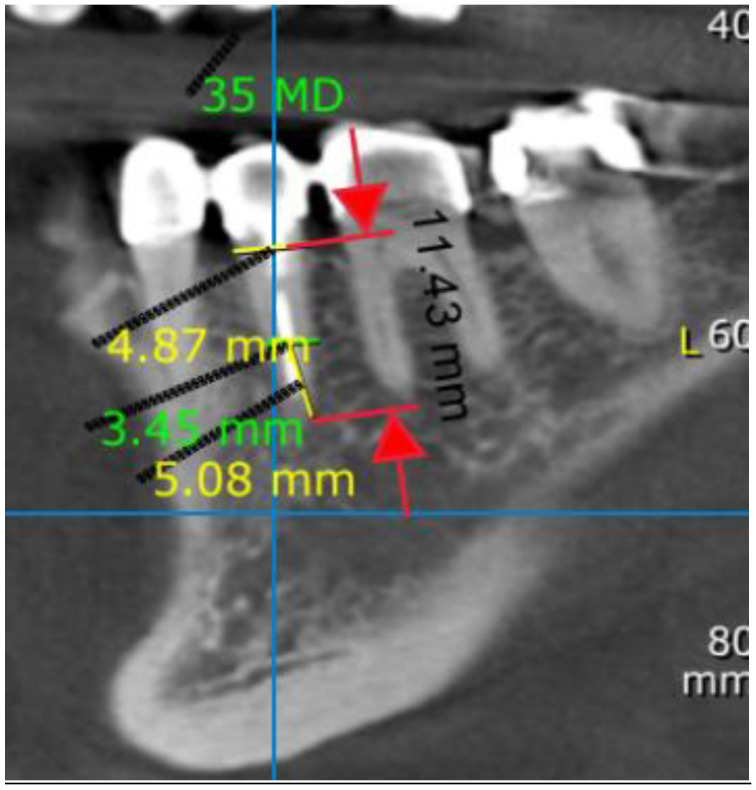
Vertical aspect of left second premolar, measured from apex to alveolar crest level; 11.43 mm in black. Mesiodistal (MD) aspect measurements, assessed in 2 points, referred to the long axis: (1) A—5 mm coronal to the apices (5.08 mm from the apex; yellow line, MD width is 3.45 mm; green line), (2) X—alveolar crest level (MD width is 4.87 mm; yellow line). CBCT para-axial sagittal section.
